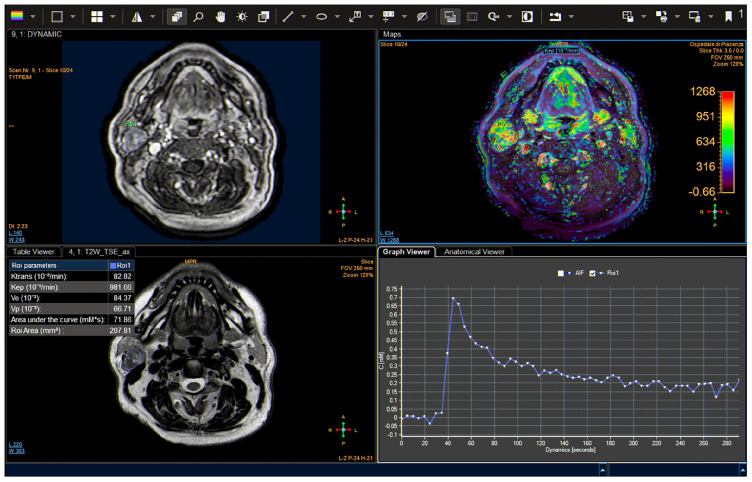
Representative multiparametric MRI findings in a Warthin tumor of the parotid gland. Multiparametric images, displayed using the clinical pharmacokinetic analysis interface, are organized as follows. (Upper left): dynamic contrast-enhanced acquisition. (Lower left): axial T2-weighted anatomical image demonstrating a solid lesion within the right parotid gland, with an overlaid table reporting quantitative pharmacokinetic parameters derived from ROI placement. (Upper right): color-coded Kep map overlaid on a contrast-enhanced T1-weighted image, showing the spatial distribution of Kep within the lesion and a measured value of 981 × 10^−3^/min. (Lower right): corresponding time–intensity curve showing rapid early enhancement followed by marked wash-out, consistent with a type B curve.
